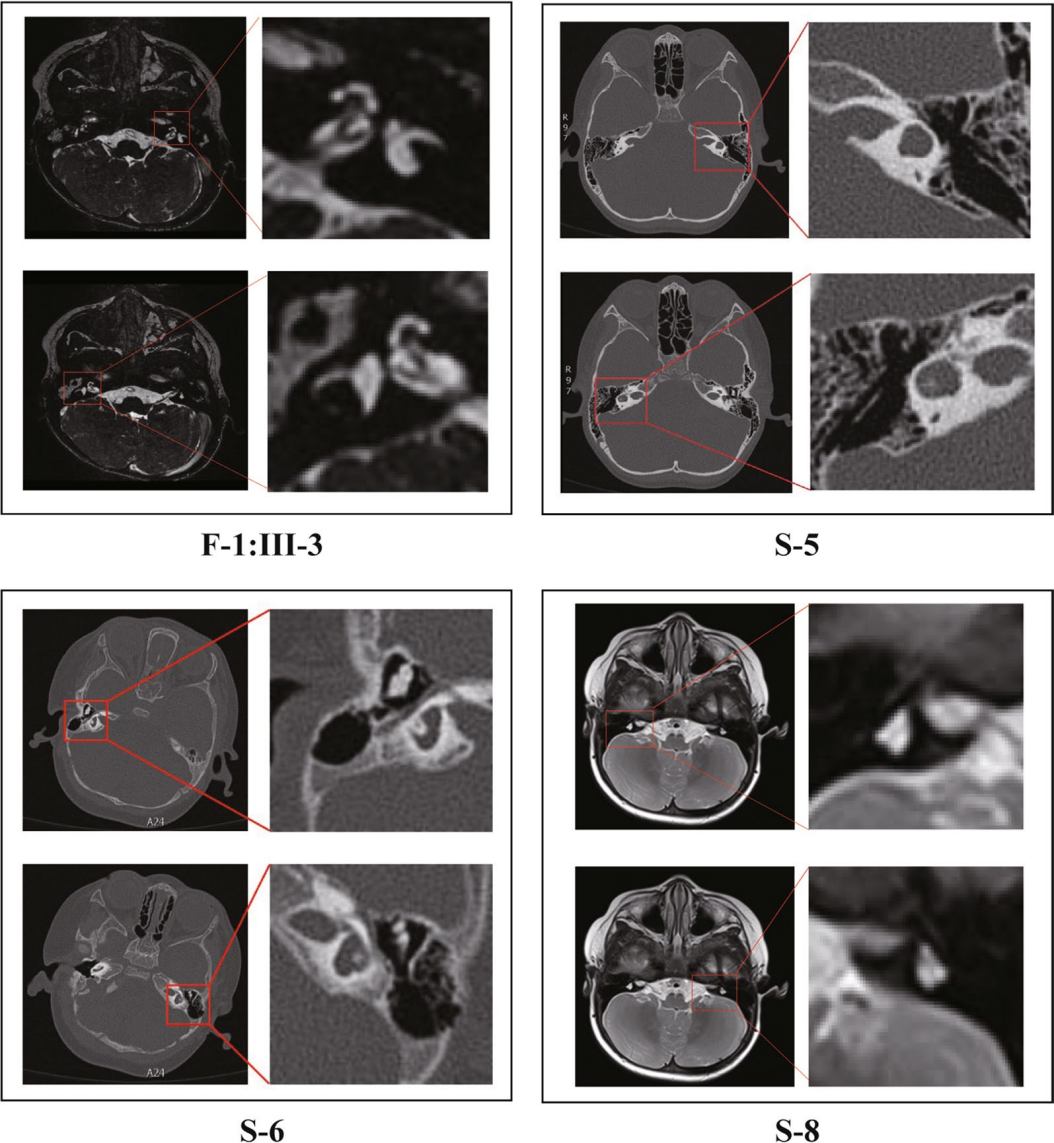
Temporal bone CT and cranial MRI of WS probands with inner ear malformation
